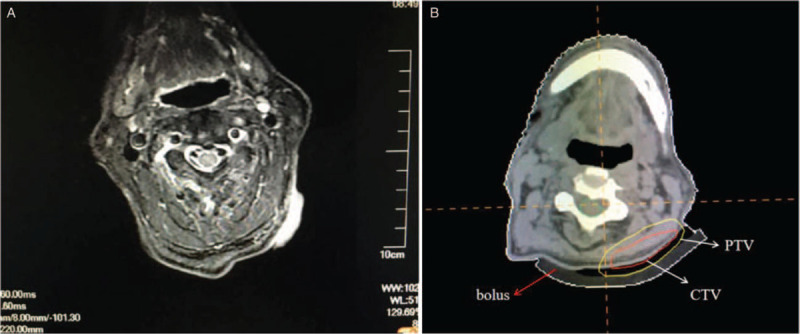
Radiotherapy target volume delineation. (A) T2-weighted magnetic resonance imaging (MRI) of the neck was used to delineate the target volume of the tumor. (B) With reference to MRI of the same anatomical section, the target volume of radiotherapy was contoured onto the computed tomography image of the treatment planning system. The red arrow-marked bolus was used to enhance the dose delivered to the skin. The red circle referred to the clinical target volume (CTV). The yellow circle referred to the planning target volume (PTV).
